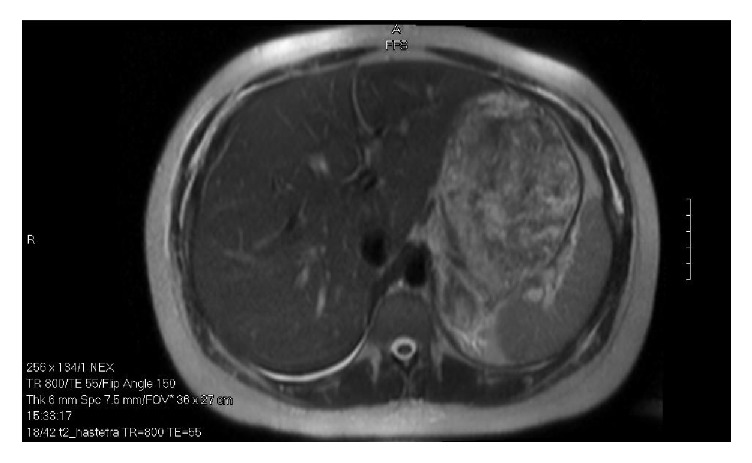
MRI findings of left adrenal hemorrhage.
